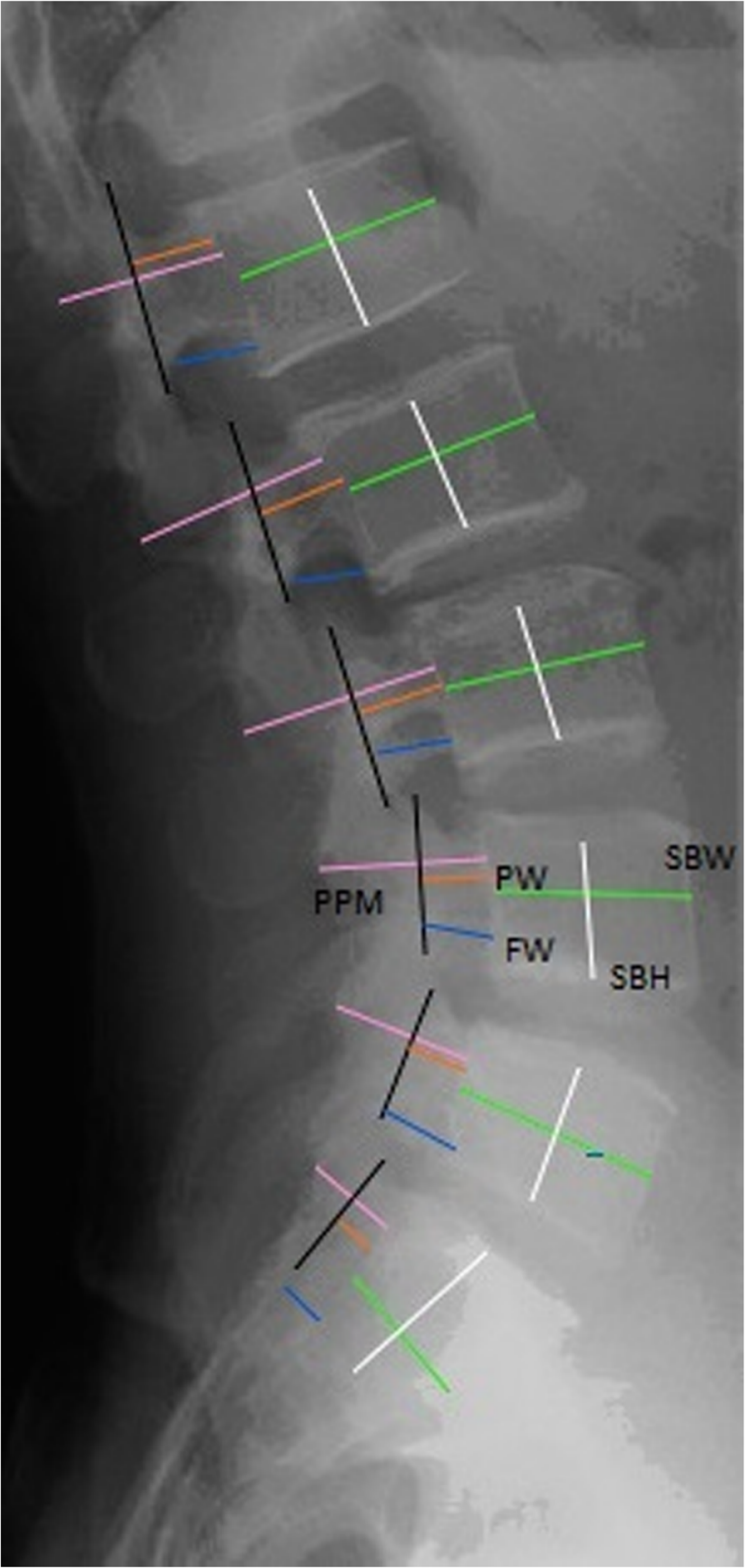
Measurement scheme for the lateral standing radiograph: sagittal vertebral body width (*SBW: green*), sagittal vertebral body height (*SBH: white*), pedicle width (*PW: orange*), posterior pedicle margin (*PPM: pink*), and foraminal width (*FW: dark blue*). The *black line* indicates how the line connecting the facet joints should be outlined to identify the posterior margin of the pedicle width
